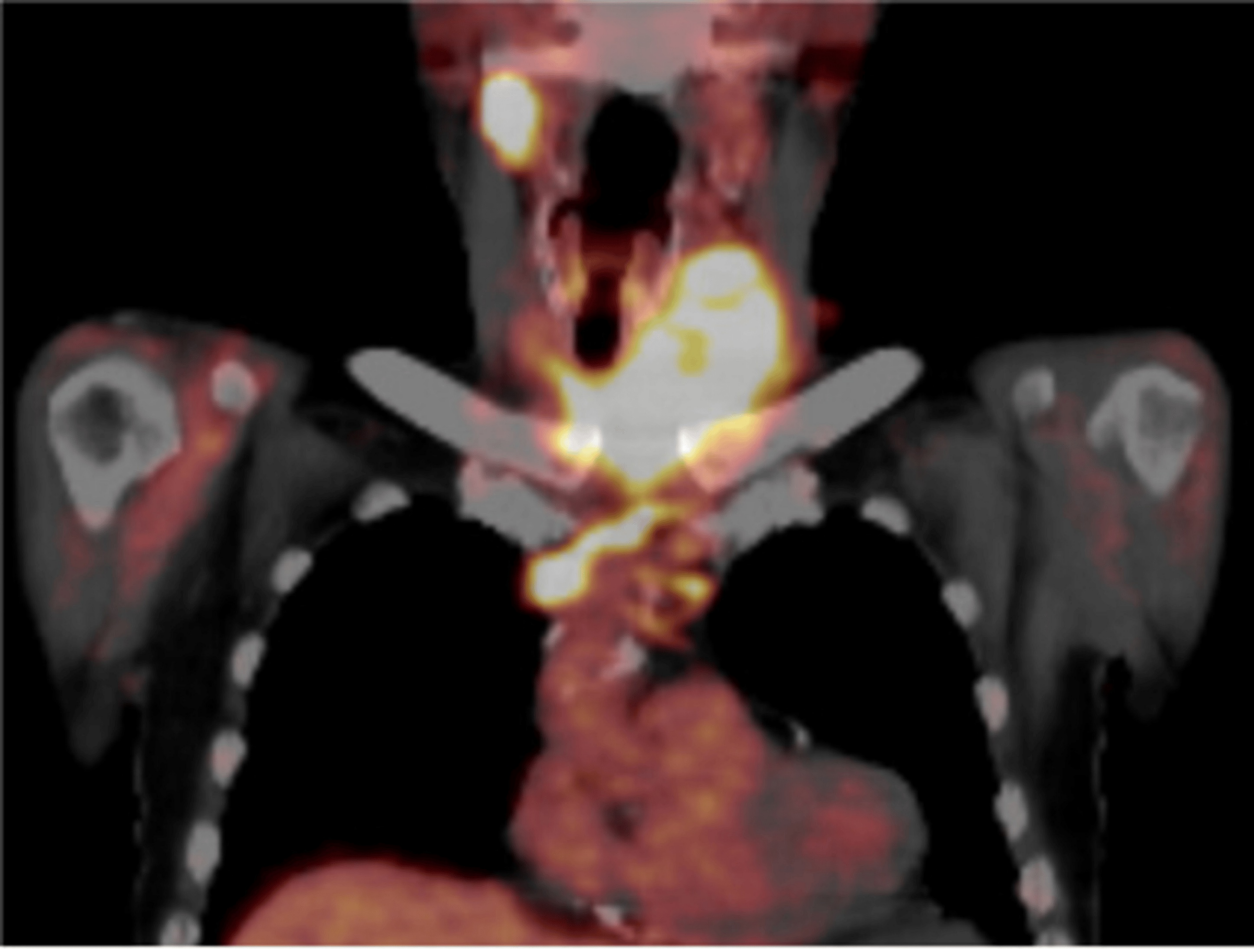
PET-CT of the thyroid tumor revealing an abnormal FDG uptake in the left thyroid lobe and in the tumor extending along the vessels, confirming intravascular invasion.
